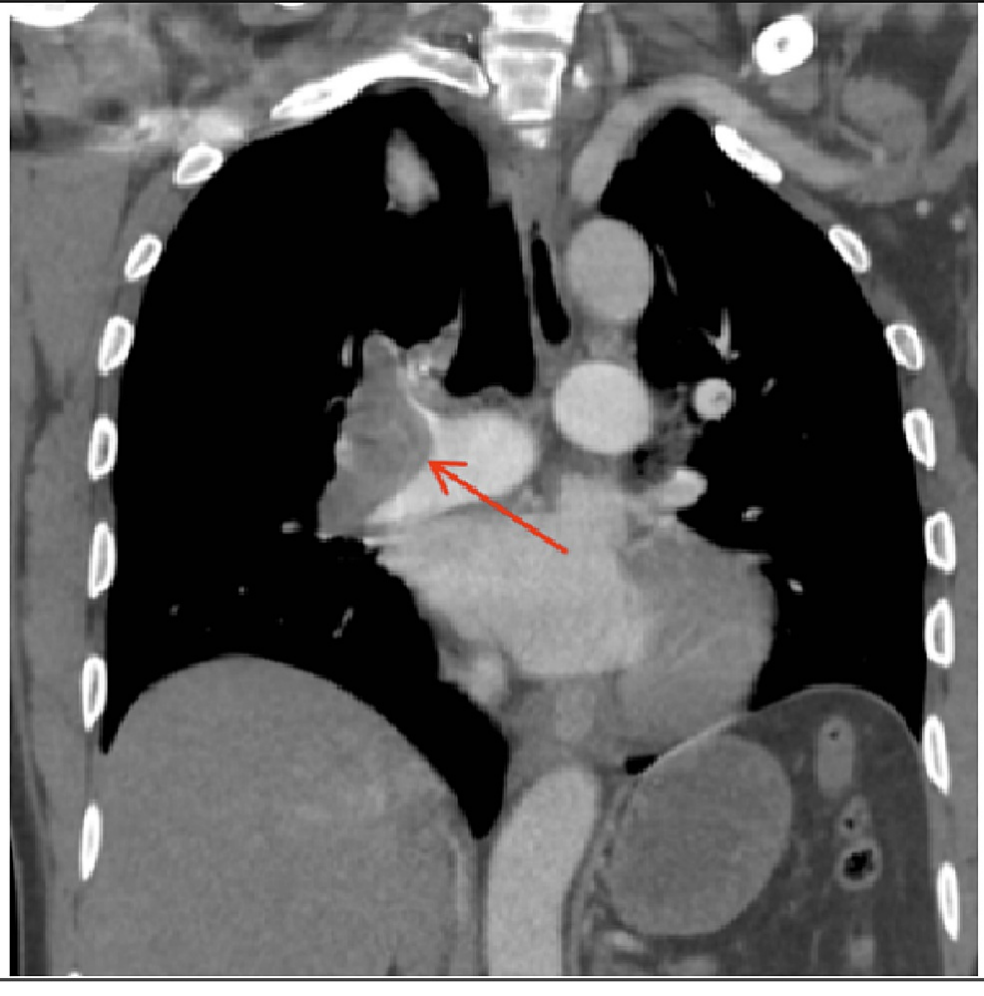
Coronal CT showing right (R) pulmonary artery embolism
